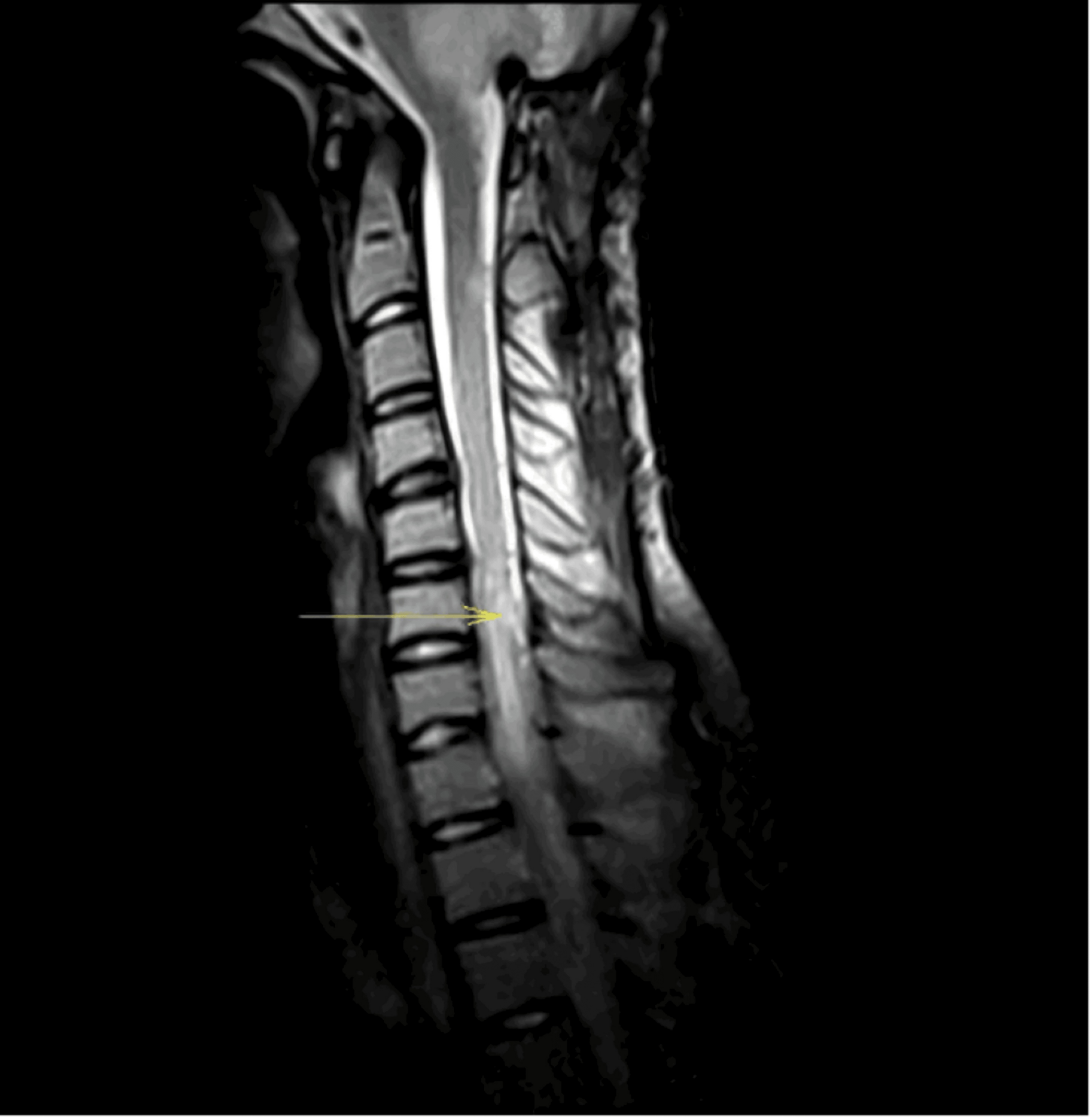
Sagittal MRI of the cervicothoracic spine demonstrating intramedullary metastatic infiltration (yellow arrow) with leptomeningeal dissemination one year after surgery.
